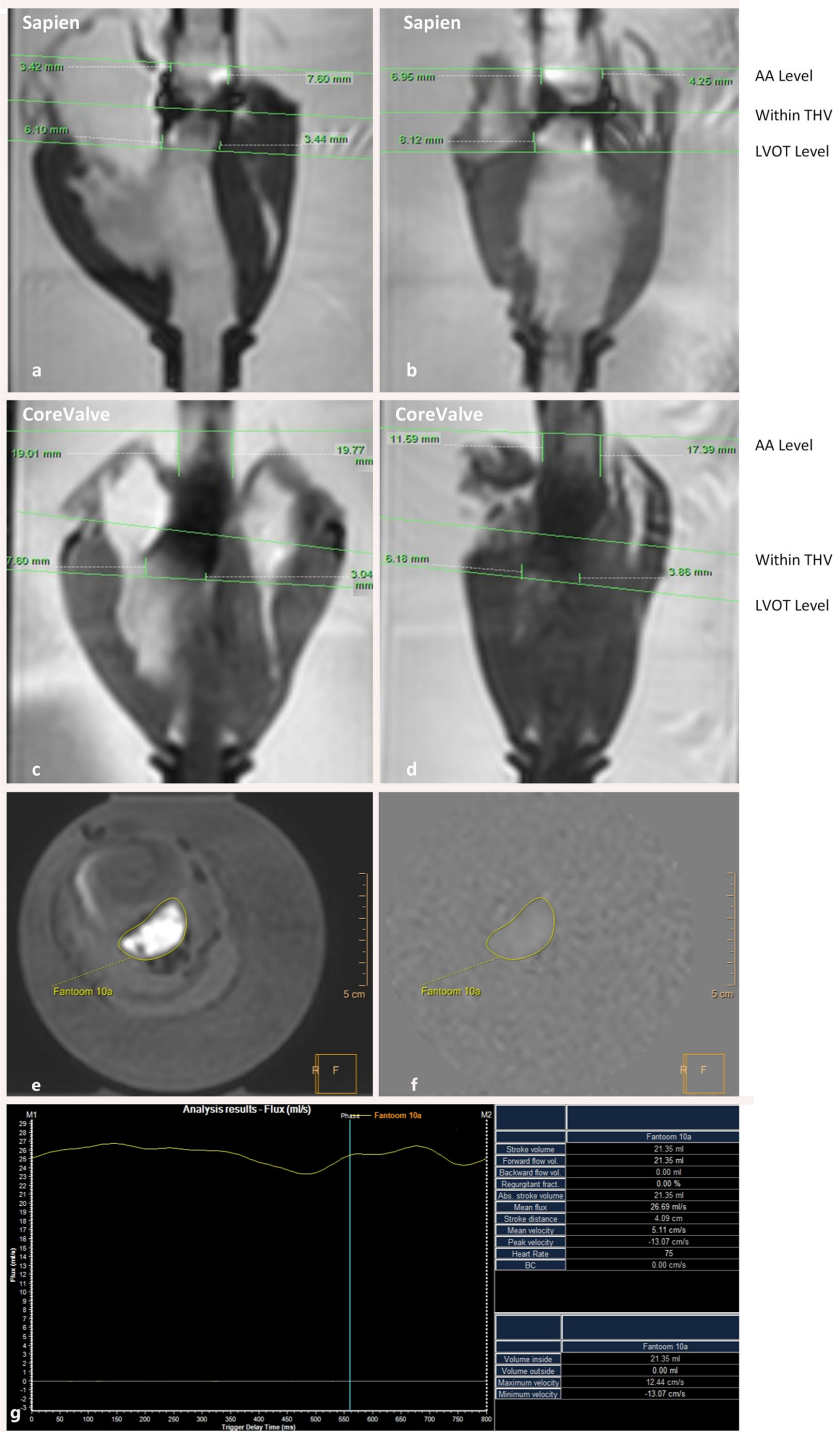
Example of flow analysis by cardiac magnetic resonance. For each THV valve, the 2 perpendicular views with plane selection per level and distance to the THV frame are shown (a–d). e Cardiac MR image of the heart at LVOT level. Yellow circle: ROI. f MR velocity–encoded image. Yellow circle: ROI. g Curve of flow during one acquisition. AA, ascending aorta; LVOT, left ventricular outflow tract; ROI, region of interest
